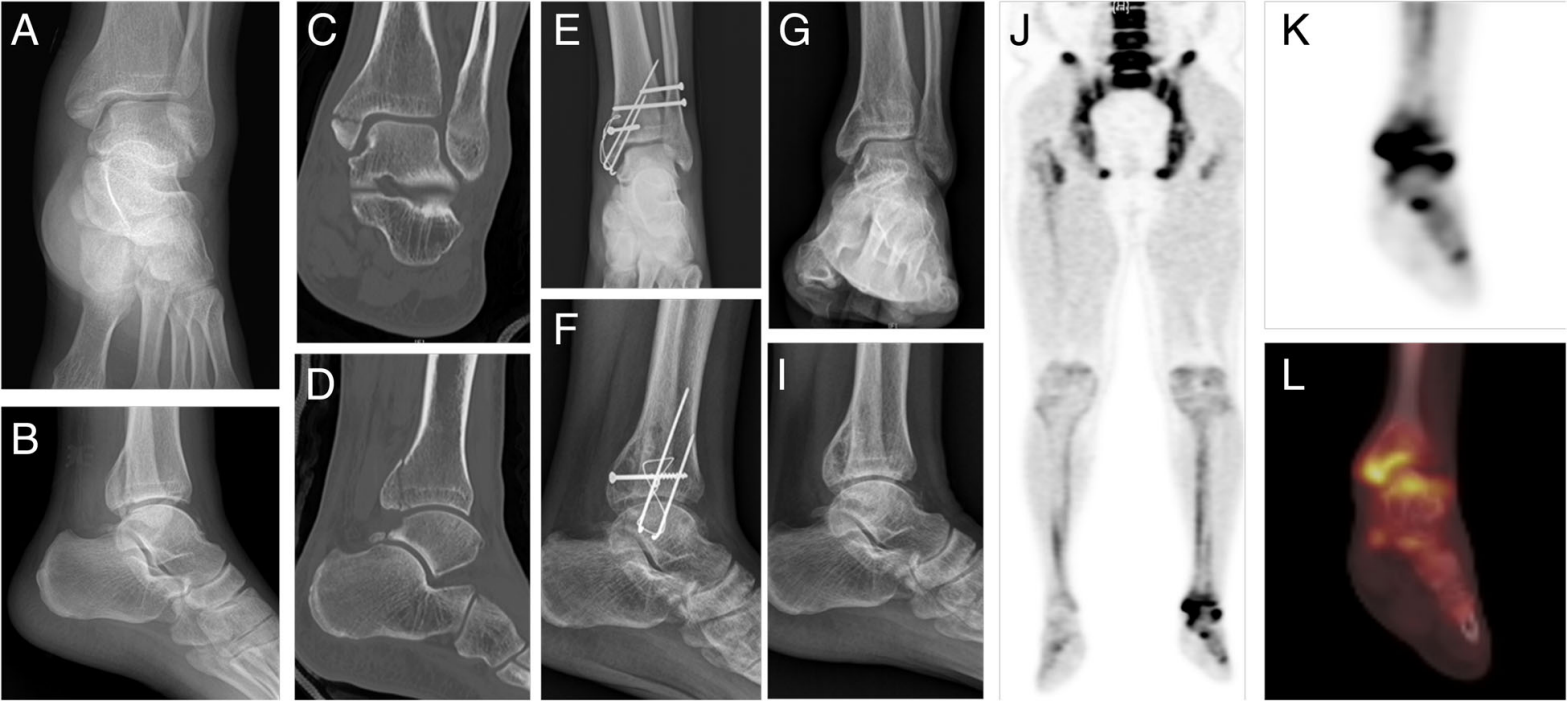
Radiological and ^18^F-Sodium fluoride (18F-NaF) positron emission tomography-computed tomography (PET/CT) images of a 37-year-old male patient who had a fracture of the left medial and posterior malleolus of the tibia. a-d Initial images of plain radiographs and computed tomography (CT); a ankle anterior-posterior view, b ankle lateral view, c ankle CT coronal view, d ankle CT lateral view. Note that the fracture involved the talocrural (tibiotalar) joint. e, f Plain radiographs after internal fixation with a metal pin. g-i Plain radiographs performed at 17 months after injury presents pin removal status and osteoporotic changes around the ankle joint, but does not provide any information about the degree of ankylosis in the ankle. j-l) 18F-NaF PET/CT shows hot uptake around the talocrural joint near the initial fracture area
